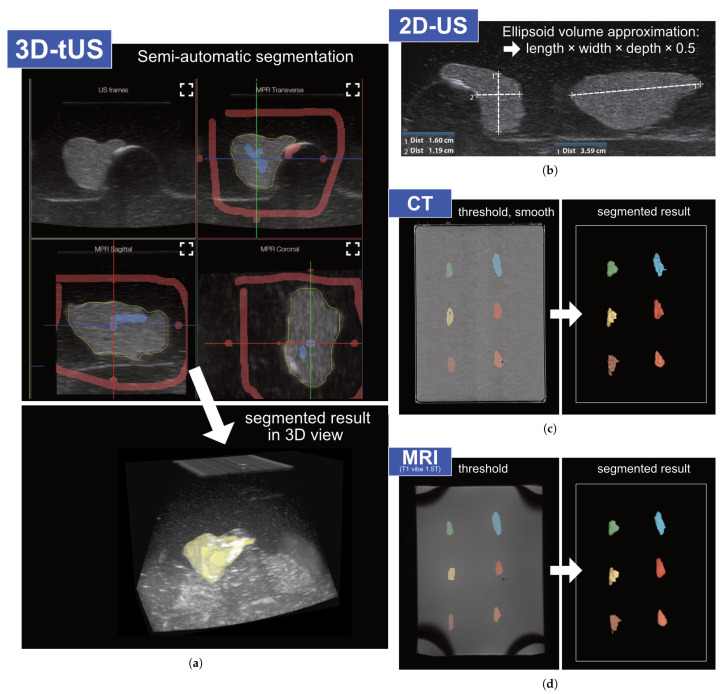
Volume measurement and segmentation process. White arrows correspond to data output. (a) Tracked 3D-US: semi-automatic segmentation of volume with binary colouring of tomographic ultrasound data within the PIUR tUS software; (b) Bmode-2D-US: measurement for volume estimation of a sample in Bmode-2D-US; (c) CT: segmentation of all samples from CT scan; (d) MRI: segmentation of all samples from MRI scan.
